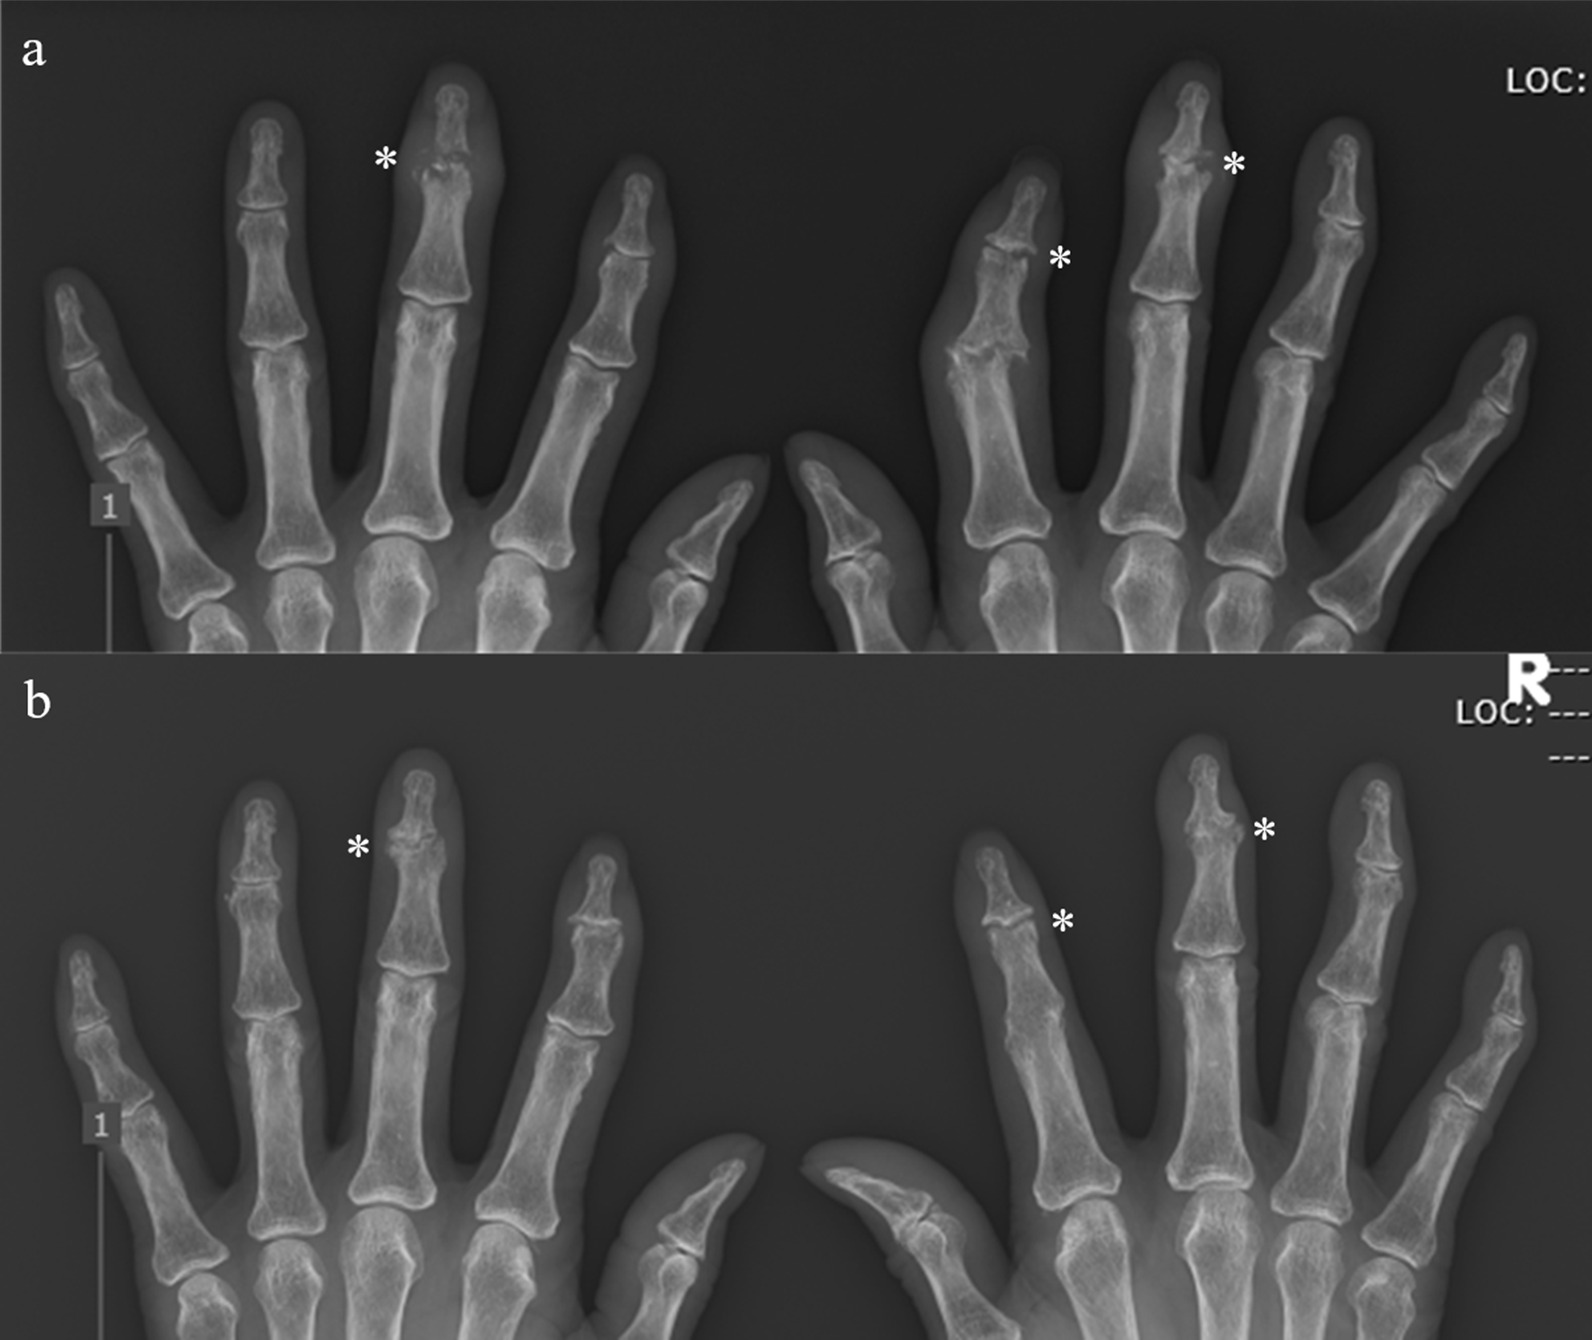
The time course of the anteroposterior radiograph of the distal interphalangeal joint (asterisk). a First visit at our hospital. b Six years of tocilizumab treatment
